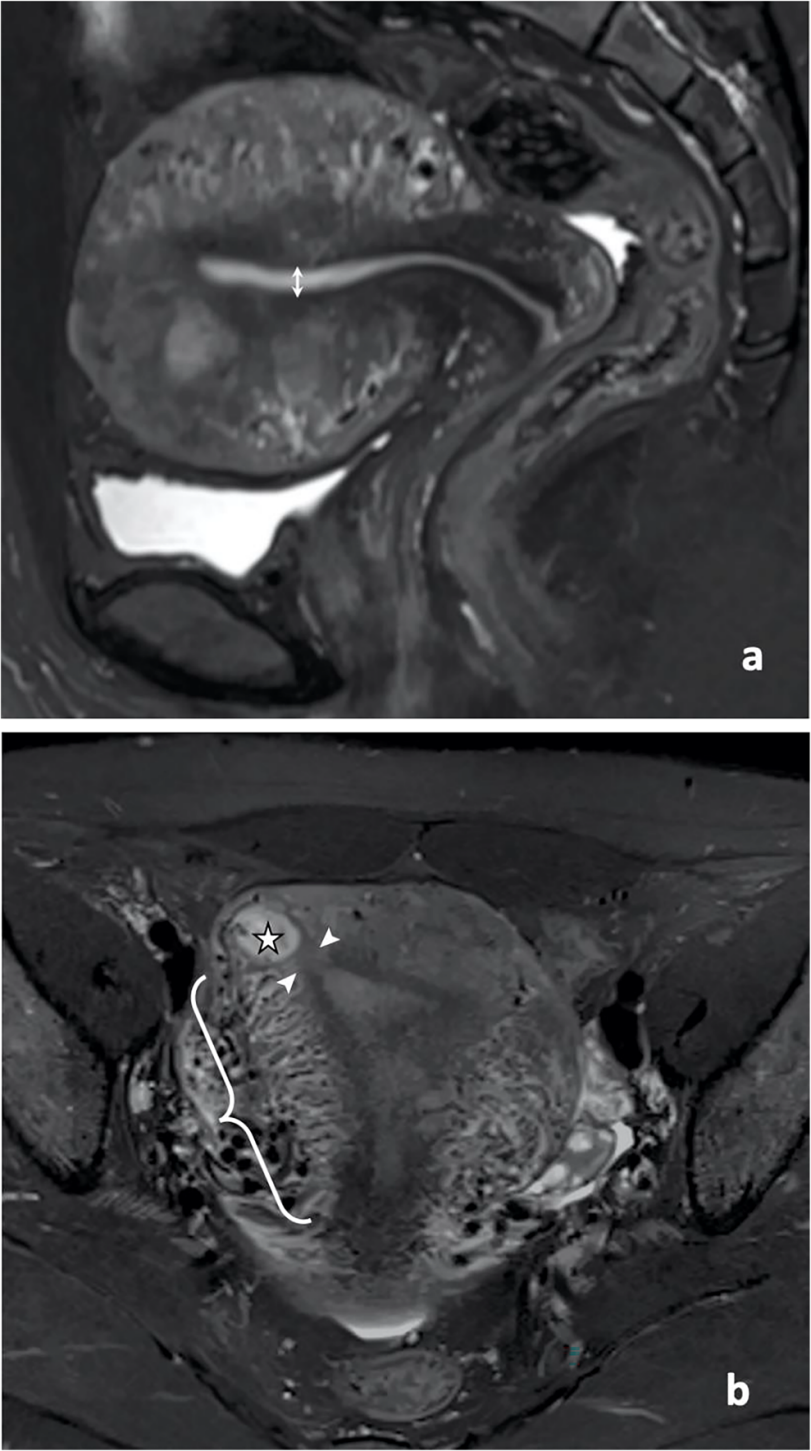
Fat-suppressed, T2-weighted MRI in a 34-year-old patient with interstitial pregnancy. a Sagittal image showing an endometrial thickness (double-ended arrow) of 3.6 mm. b Coronal image showing the gestational sac (star), intact lateral junctional zone (arrowheads), and ipsilateral flow-void (curly bracket)
